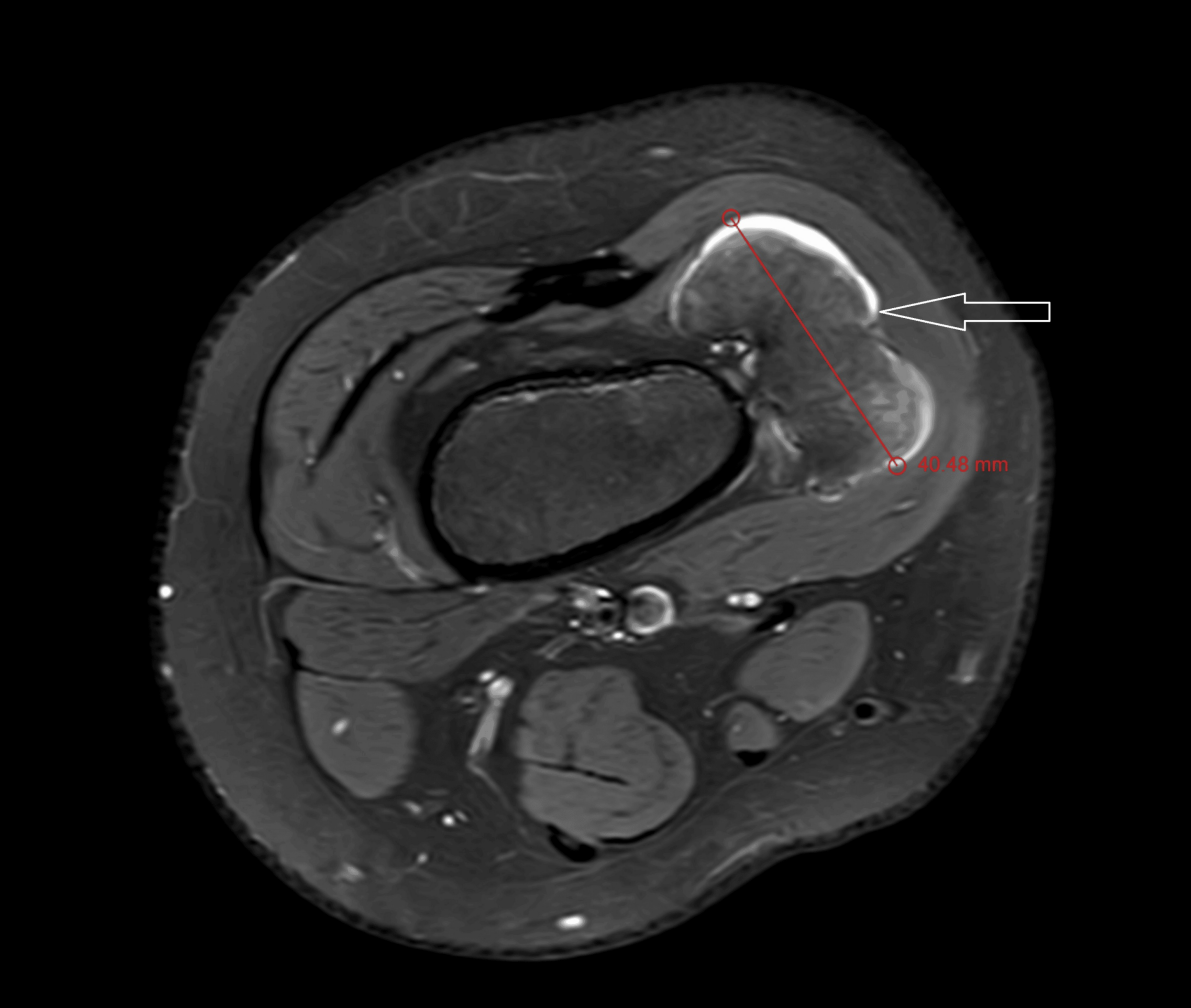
The axial PD fat-suppressed MRI shows the anteroposterior dimension of the sessile outgrowth and the continuation with the parent bone. PD fat sat: proton density fat suppression image
